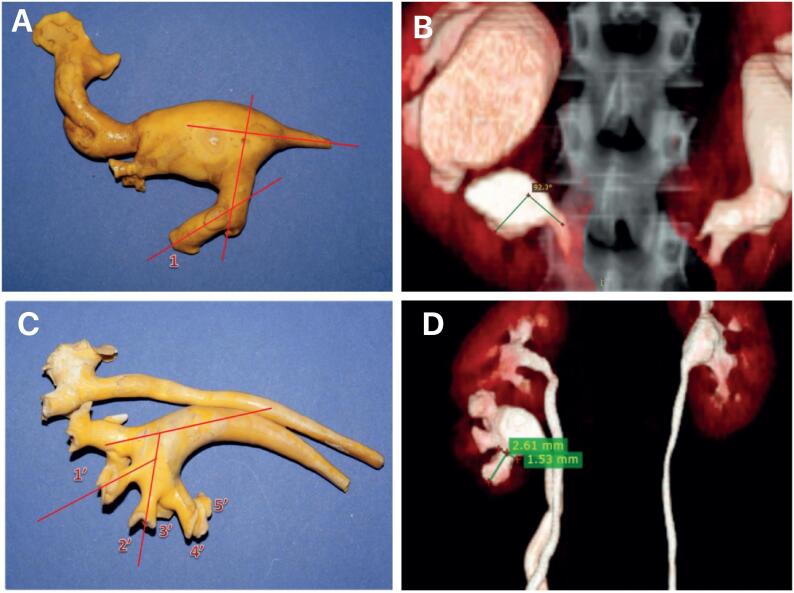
Example of the measurements performed in this study with a computer program (15). A) The figure shows an endocast of a horseshoe kidney. The angles between the lower infundibulum and renal pelvis (LIP) are measured; B) The figure shows the computed tomography (CT) scan image of one of the horseshoe kidneys analyzed showing the measurement of the LIP; C) The figure shows an endocast of a Complete Ureteral Duplication (CUD) showing the angles measurements and D) The figure shows the CT scan image of one of the CUD analyzed showing the measurement of the length and width of the infundibulum of the lower unit.
